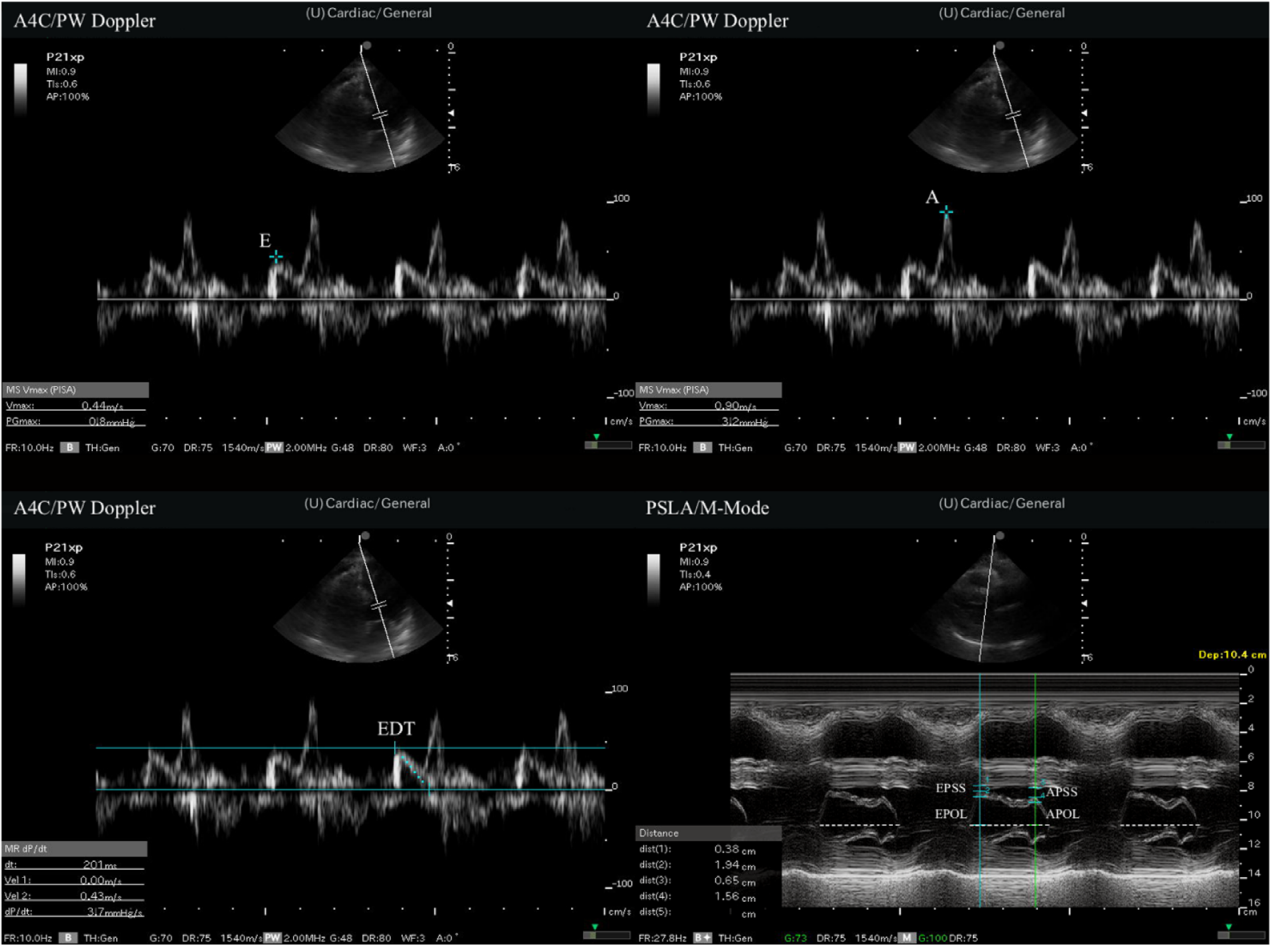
Measurements of point-of-care ultrasound. *A*, late diastole velocity of the mitral inflow; *A4C*, apical 4-chamber; *APOL*, A-point opening length; *APSS*, A-point septal separation; *E*, early diastole velocity of the mitral inflow; *EDT*, E deceleration time; *EPOL*, E-point opening length; *EPSS*, E-point septal separation; *PSLA*, parasternal long axis; *PW*, pulsed wave.
